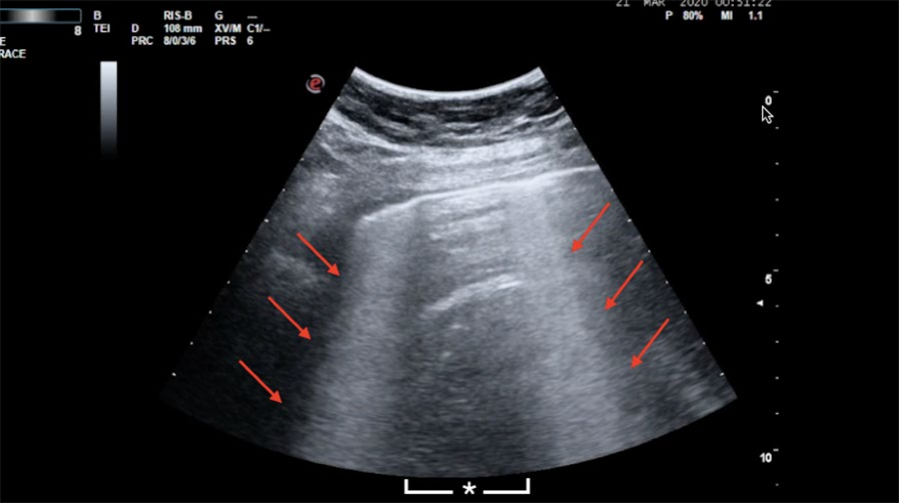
Sonographic appearance of “light beam” artifact. Red arrows indicate band-form signs arising from a large portion of a regular pleural, called “light beam”. The asterisk indicates the spared area (A-lines). Image kindly provided by Dr. Giovanni Volpicelli
